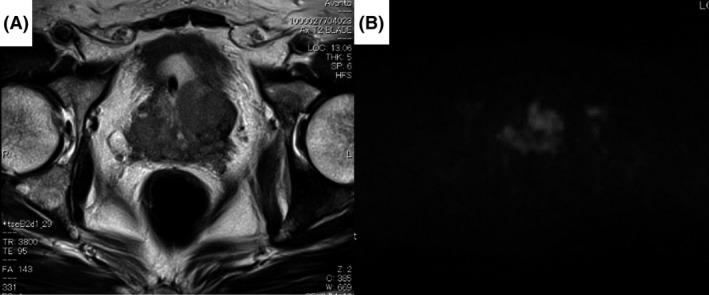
MRI (A) T2WI and (B) diffusion‐weighted image. MRI showed T2 low and DWI high‐intensity area at bilateral lobes of prostate and seminal vesicle. It suggested that prostate cancer infiltrated seminal vesicle.
